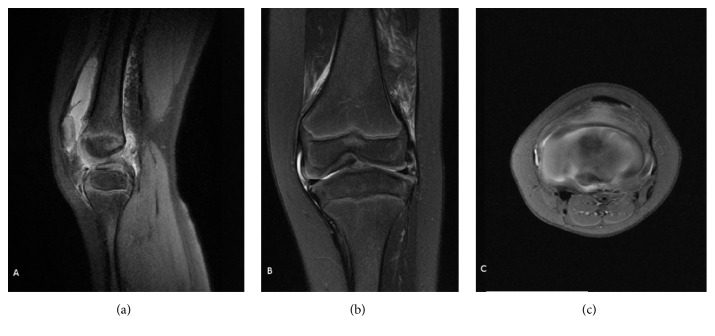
Preoperative MRI of the left knee of the patient from Figure 1 (sagittal (a), coronal (b), and transverse (c) views). Complete agenesis of the ACL and PCL hypoplasia is noted. The femoral intercondylar notch is completely covered by cartilage. The tibial spine is hypoplastic.
